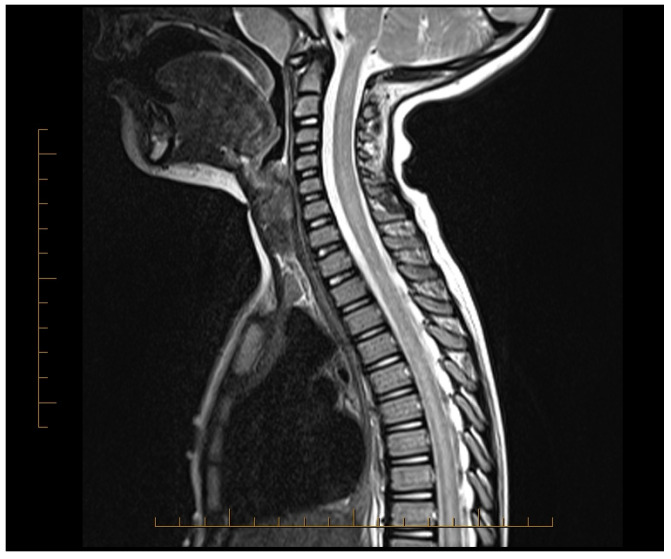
MRI of the spinal cord showing abnormal T2 hyper-intense signal throughout the spinal cord prior to initiating treatment.
